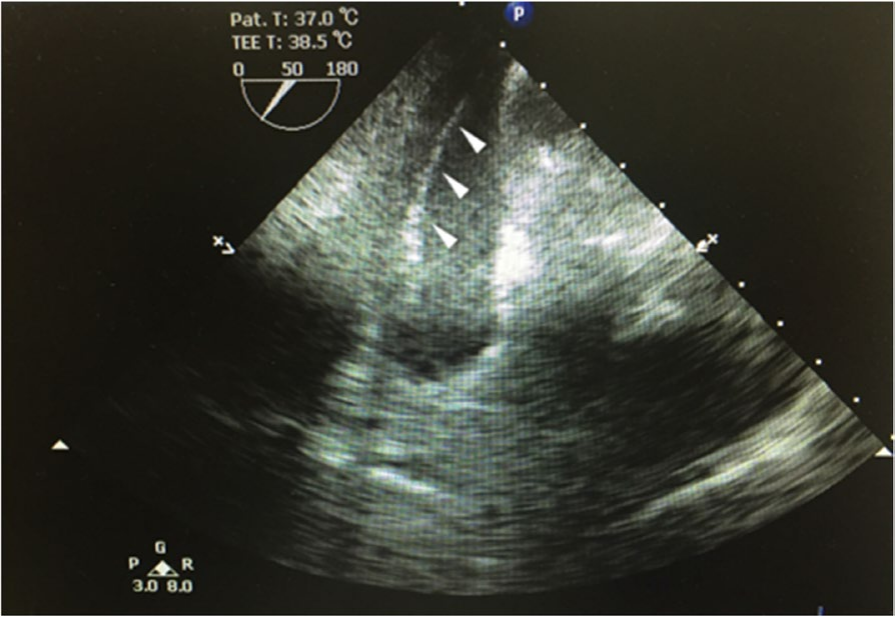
The fractured catheter was identified into coronary sinus by intraoperative transesophageal echocardiography (arrows)
